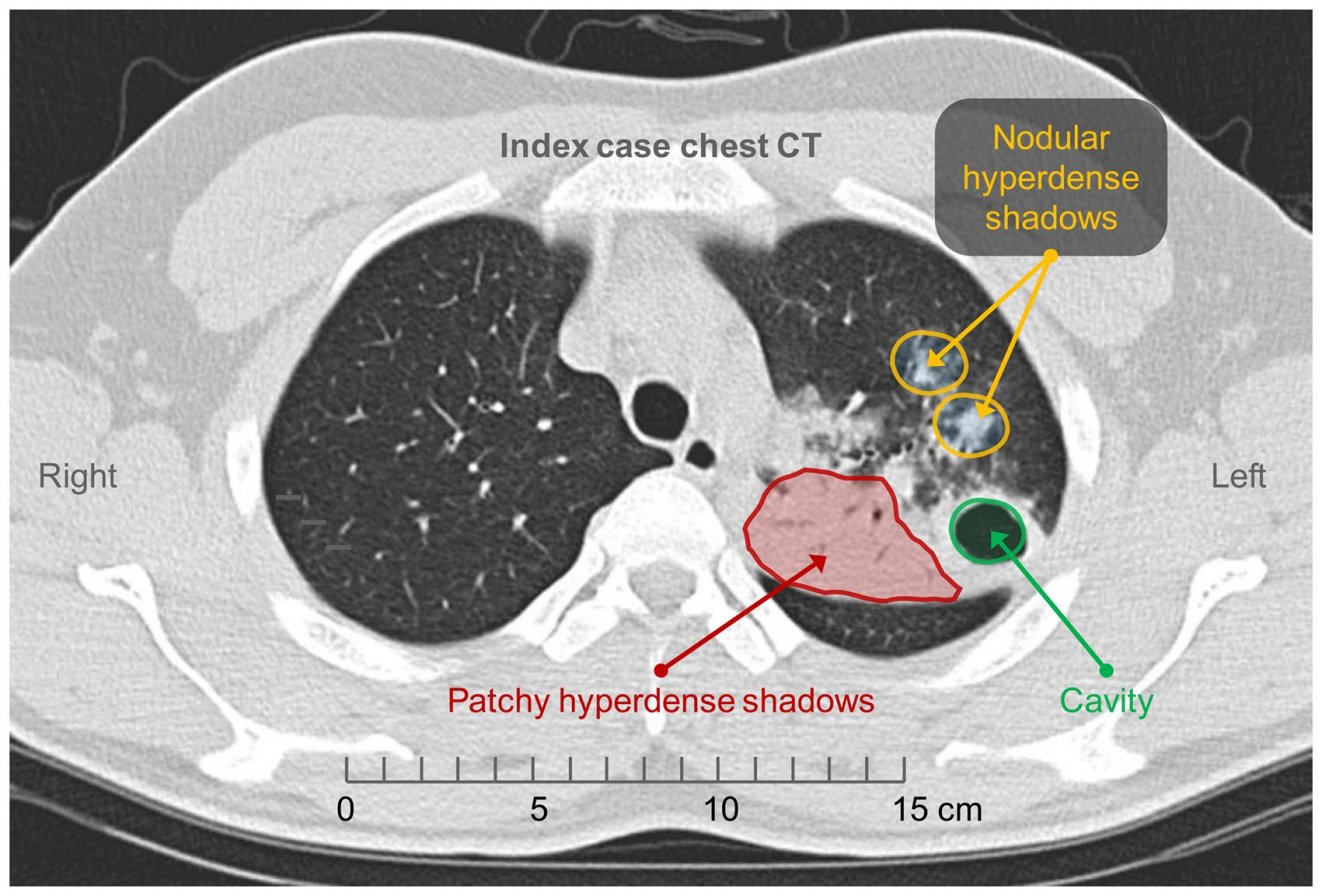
Chest CT of the index case. Patchy and nodular hyperdense shadows with cavity formation in the upper lobe of the left lung.
